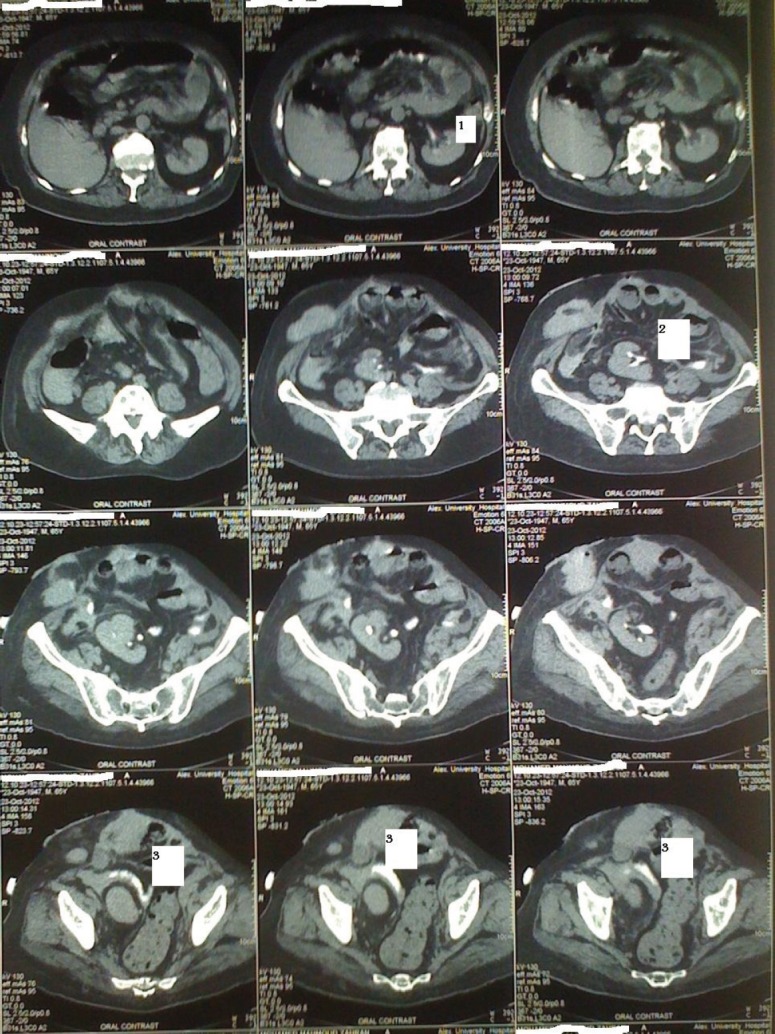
Figure 6: Postoperative CT findings. Dye is present in the conduit filling. The right and left pelvicalyceal systems: 1. Dye in the left kidney; 2. dye in the right kidney; 3. ‘Z’ conduit, passing above right kidney, and descending between the kidney and sigmoid colon.
